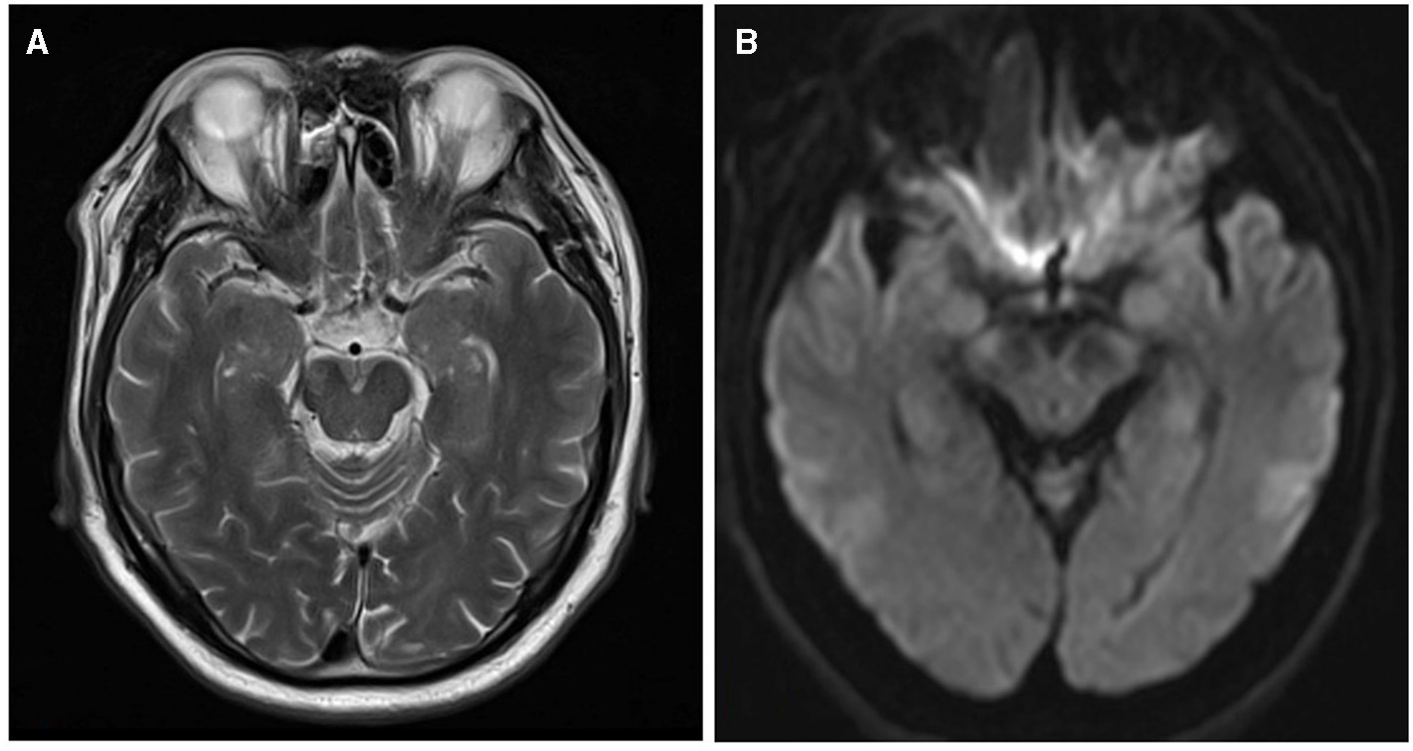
The layers on T2WI and DWI. (A) MCA-M1's normal flow void on T2WI. (B) The same layer on DWI is considered level 0.
